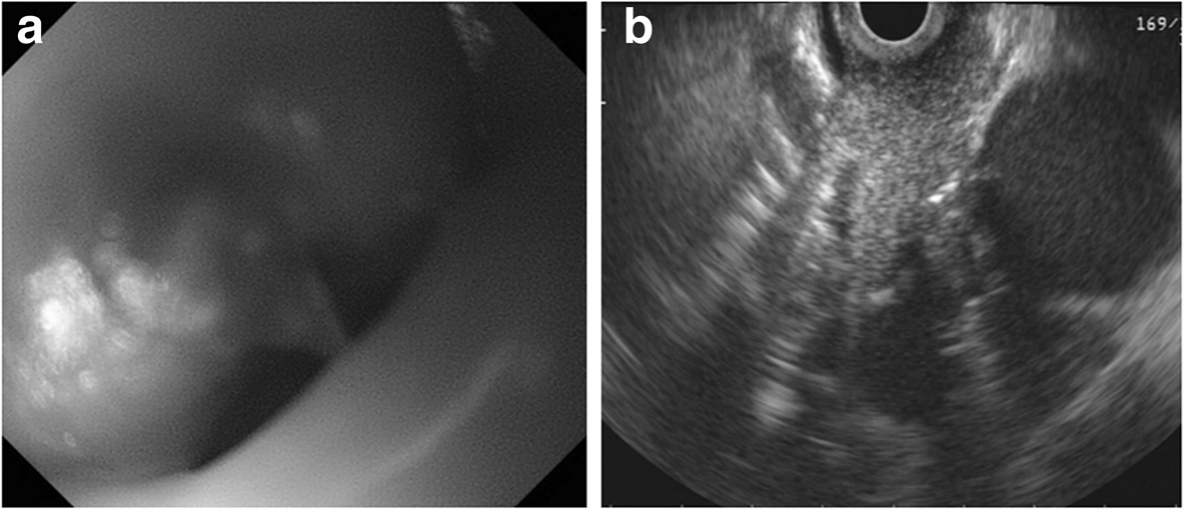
Gastrofiberscopy and fine-needle aspiration cytology. (a) Gastrofiberscopy revealed a submucosal lesion of the middle esophagus. (b) Fine-needle aspiration cytology and a boring biopsy of the esophageal lesion were obtained under ultrasonic monitoring.
